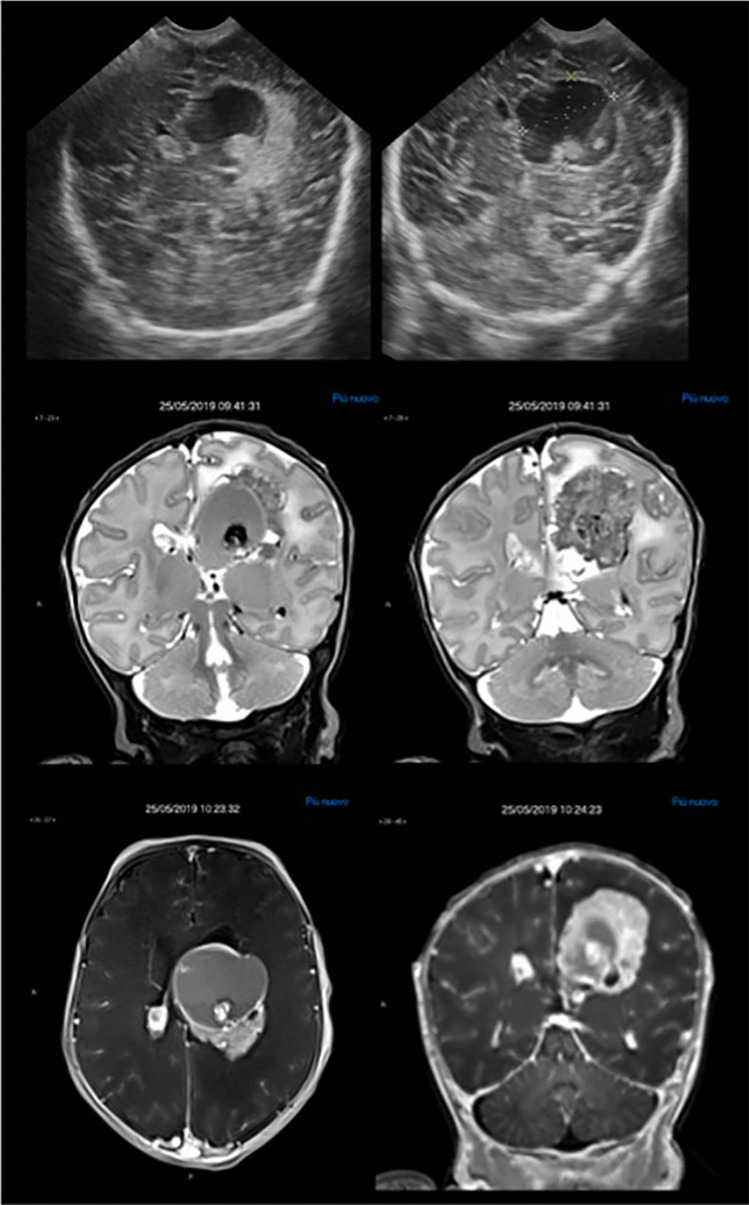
Choroid plexus carcinoma* in a newborn. Neonatal brain US images show a hyperechoic, heterogeneous intraventricular mass with blood-fluid levels expanding the left lateral ventricle (upper row). A vascular solid-cystic mass with ill-defined margins, blood products and surrounding brain edema is confirmed at MRI (lower rows). Enlargement of the choroid plexus of the lateral ventricles due to both infiltration and congestion is also noted
